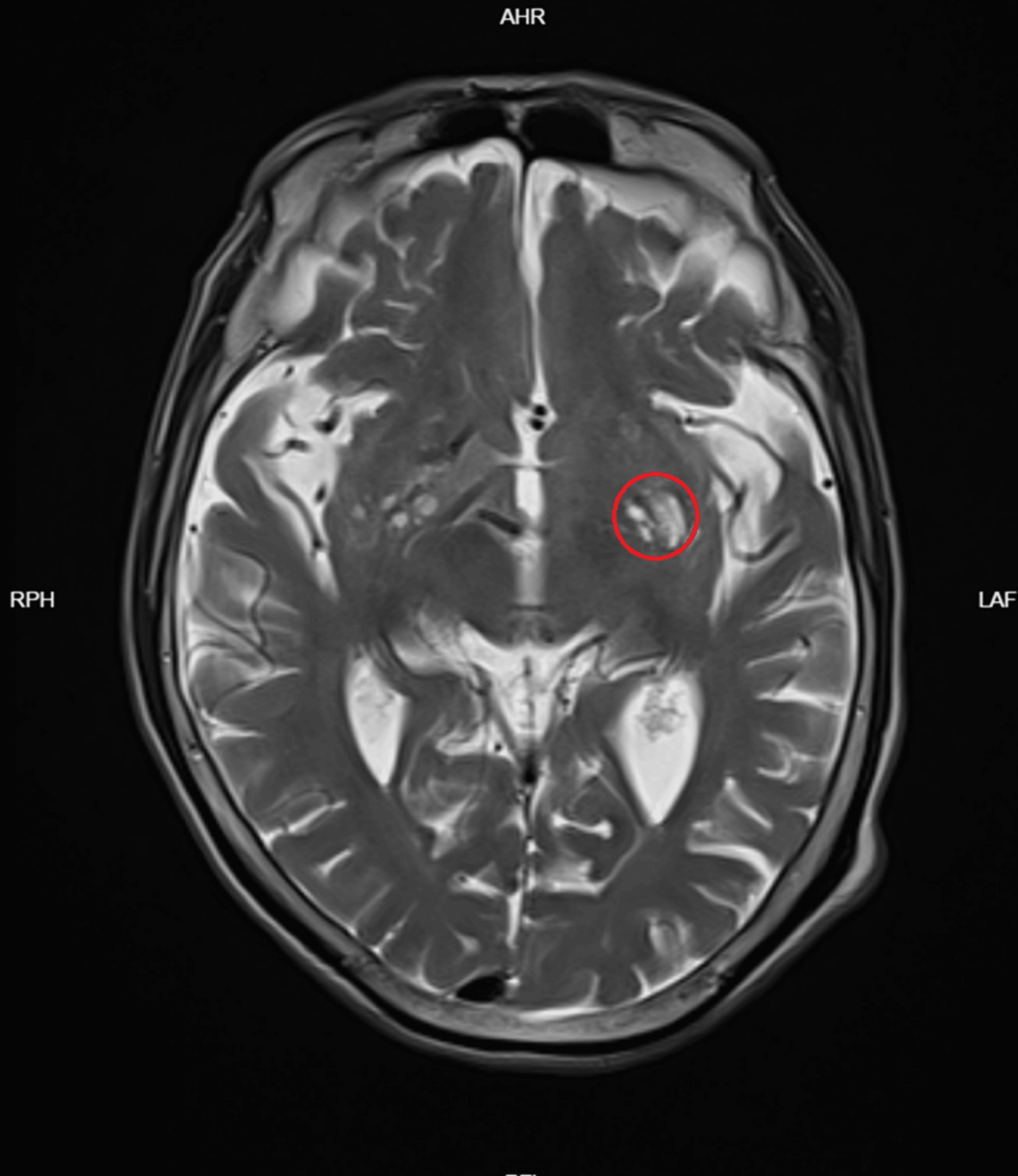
MRI T2WI demonstrating left insular softening The red circle shows a chronic lesion, excluding an acute stroke. T2WI - T2-weighted imaging
